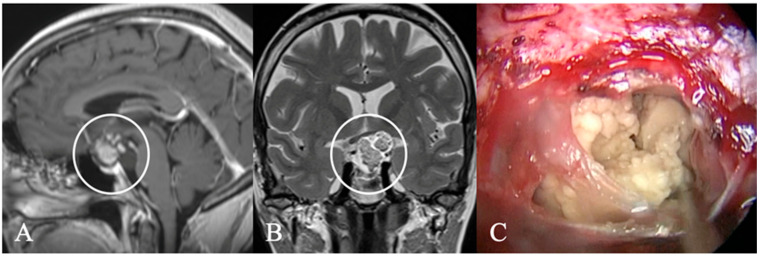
Epidermoid of a 72-year-old female patient. Initially, a visual impairment led to the diagnosis, which regressed immediately after surgery. (A) Sagittal contrast-enhanced and (B) T2 MRI shows an unusual heterogenous contrast-enhancing intra- and suprasellar mass. Intraoperative imaging (C) shows the typical white pearl-like tumor. In our case, complete resection via endoscopic endonasal approach was possible with straightforward reconstruction of the sellar using a fat pad, and no recurrence has been observed five years after the surgery.
